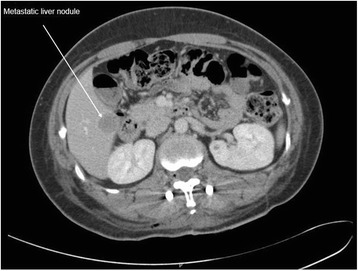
Liver metastatic nodule on initial CT
